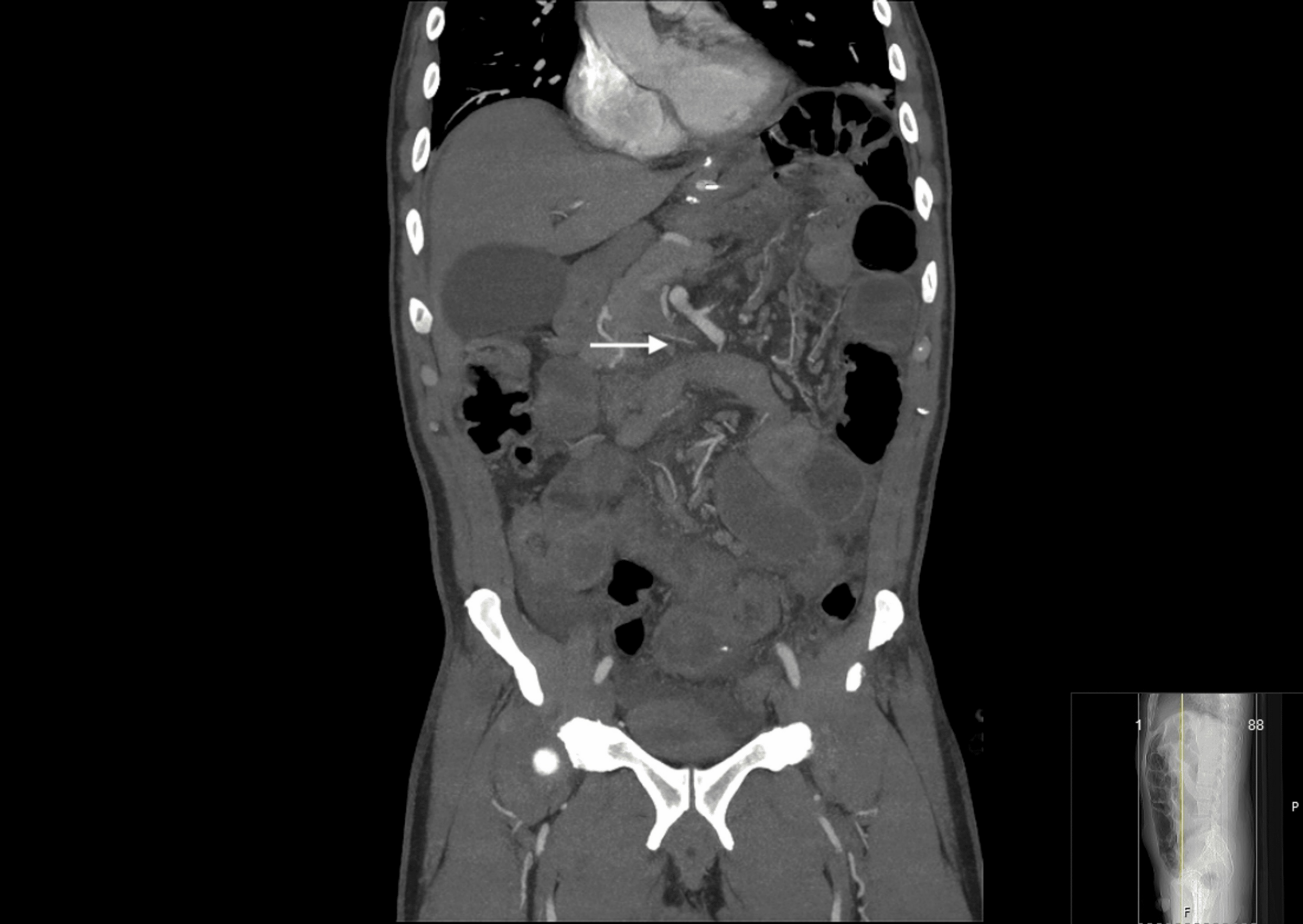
Postoperative CT scan Arrow: Resolution of the mesenteric rotation
